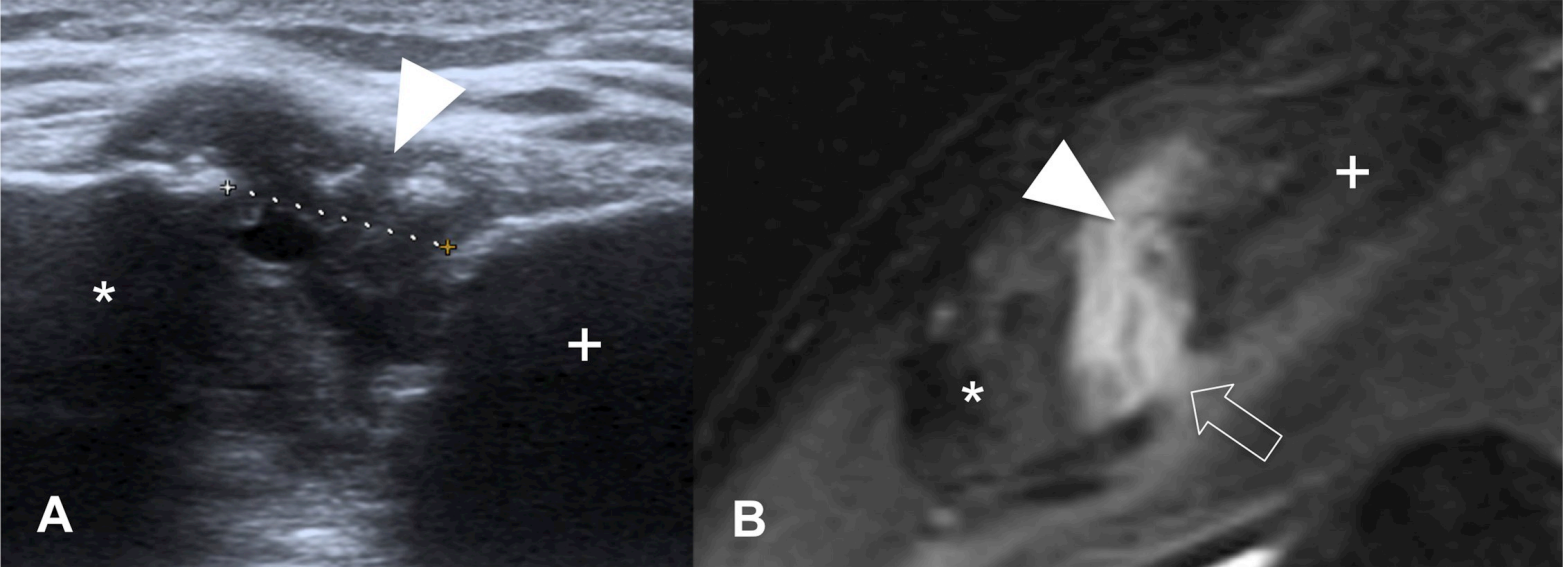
Ultrasound (A) and coronal fat saturated proton density MRI (B) of the right acromioclavicular joint in a 36-year-old male after a bicycle accident. In both modalities, the superior ACL (arrowhead) is completely absent. There is also a rupture of the inferior ACL (open arrow), which can only be seen on MRI. Joint effusion is present; acromion (*); clavicle (+).
